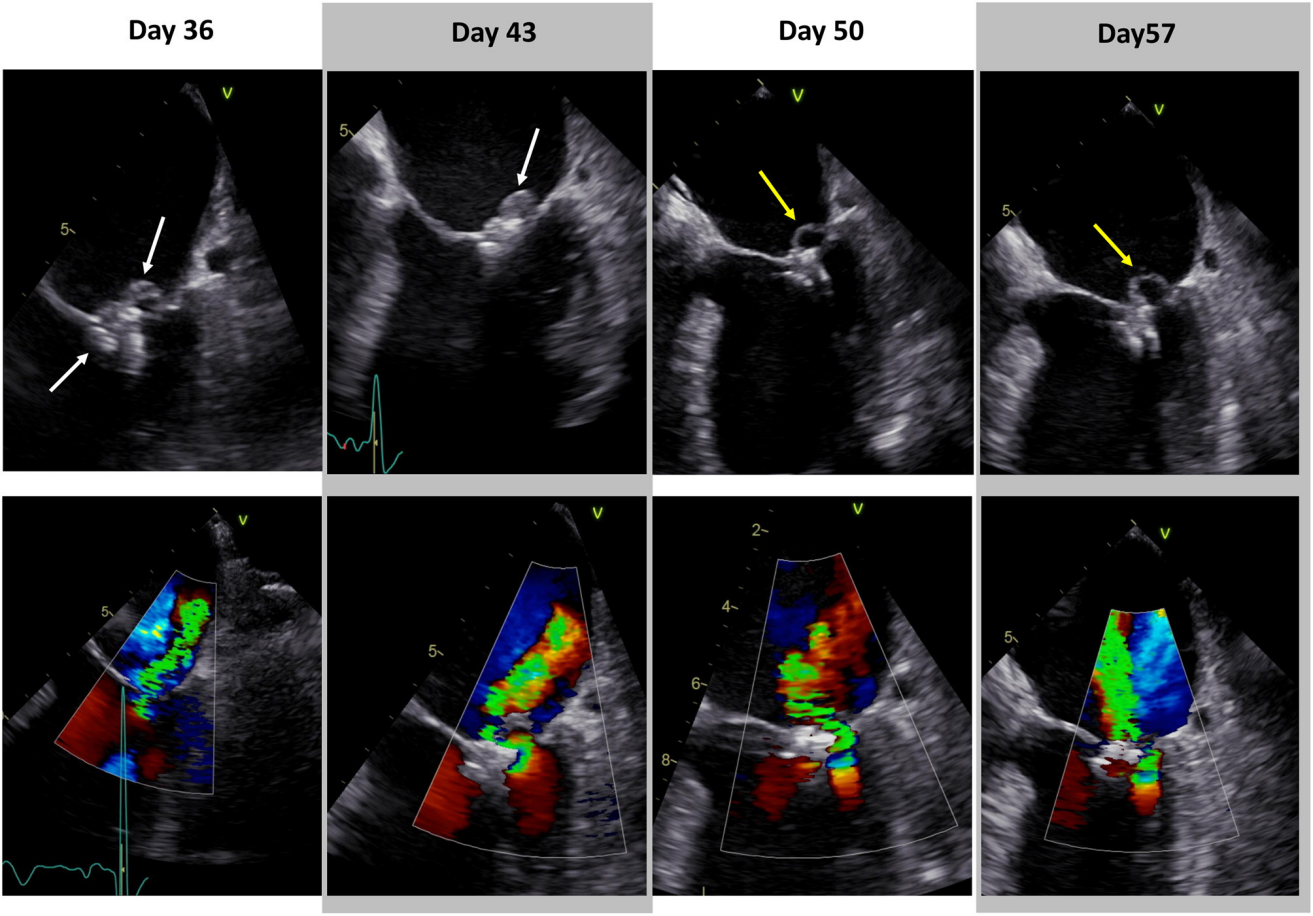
Progress of infective endocarditis in transesophageal echocardiography. Transesophageal echocardiography of infective endocarditis at various timepoints during calculated antibiotic therapy. Day 0 = transcatheter mitral valve edge-to-edge repair. White arrows indicate vegetations. Yellow arrows show perforated posterior mitral leaflet and significantly reduced vegetation (Day 36: Supplementary Videos 3, 4; Day 43: Supplementary Videos 5, 6; Day 50: Supplementary Videos 7, 8; Day 57: Supplementary Videos 9, 10).
